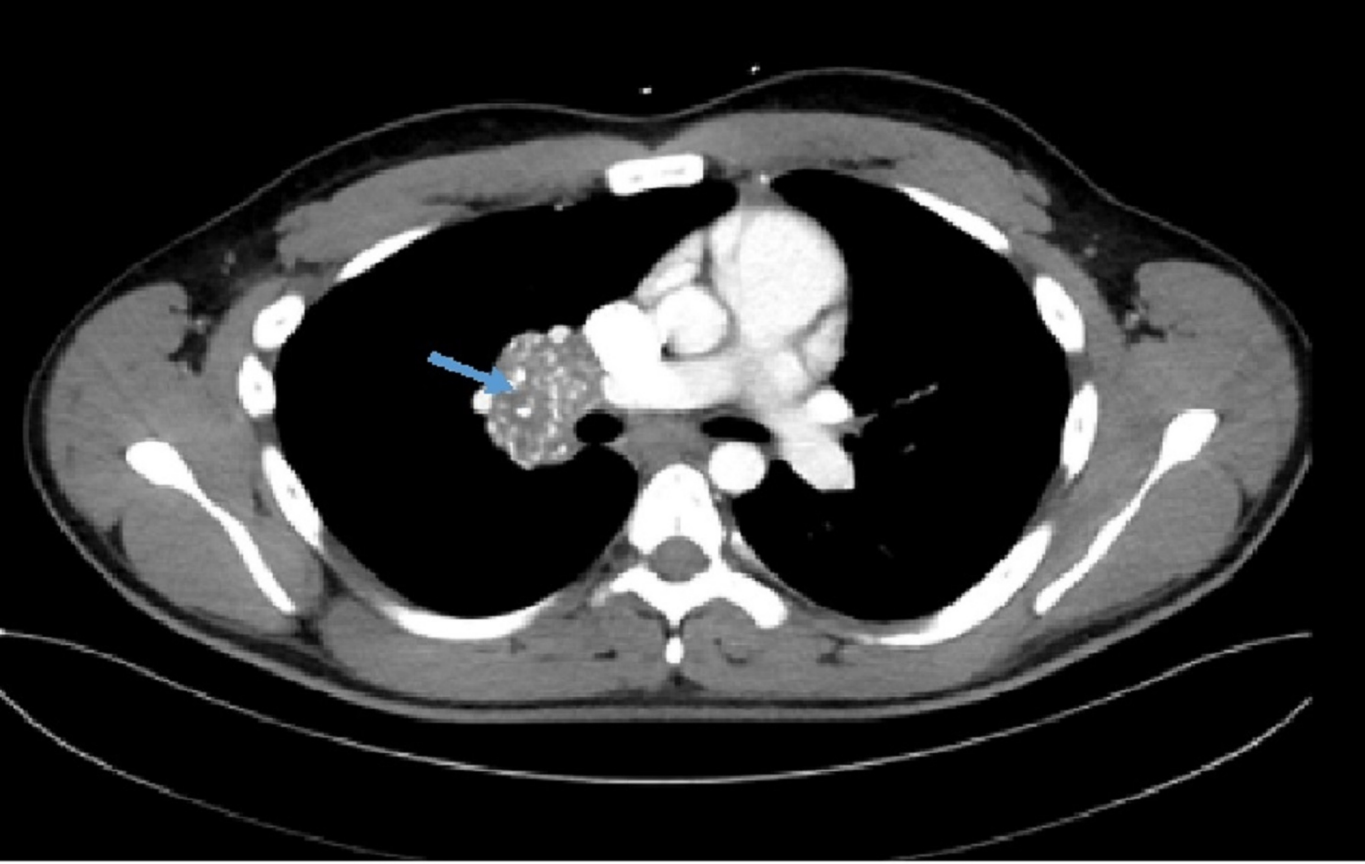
Computed tomography (CT) chest showed perihilar mass (Arrow).
